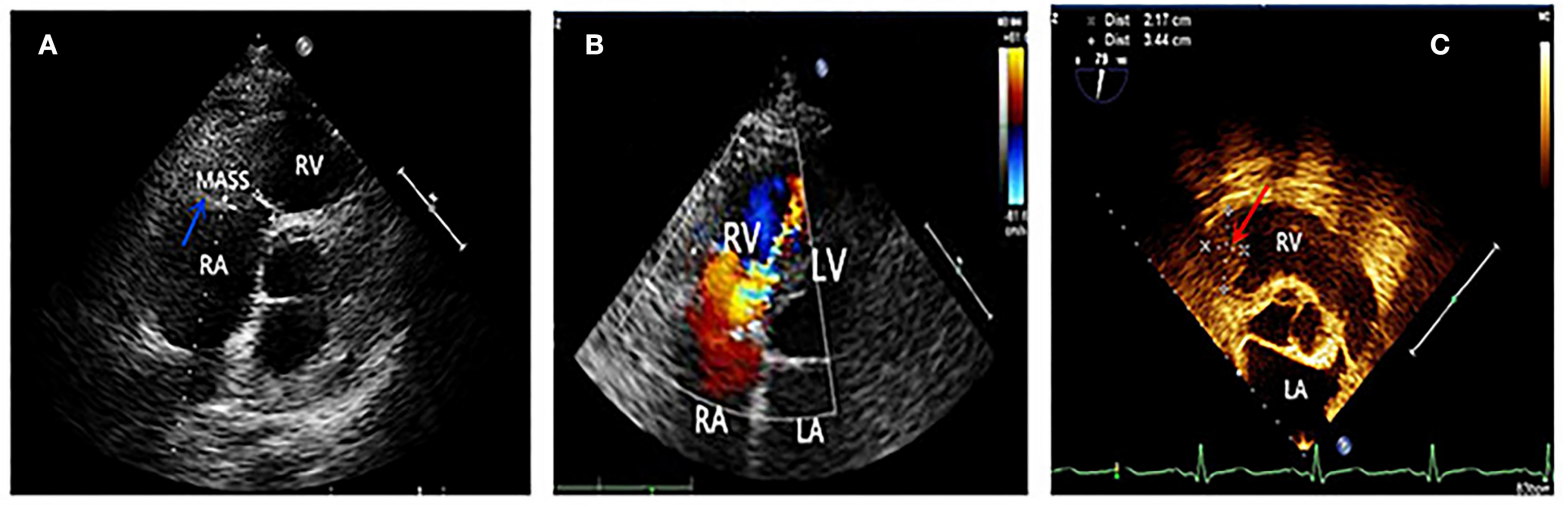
(A) Blue arrow showed a mass in the right ventricle, which was later confirmed thrombus by surgery. (B) Four chamber view showed the mosaic blood flowing through the stenotic right ventricular inflow tract. (C) Two and a half years after tricuspid valve replacement and thrombectomy, a recurrence of thrombus (indicated by red arrow) was seen in the right ventricular inflow tract by transesophageal echocardiography.
